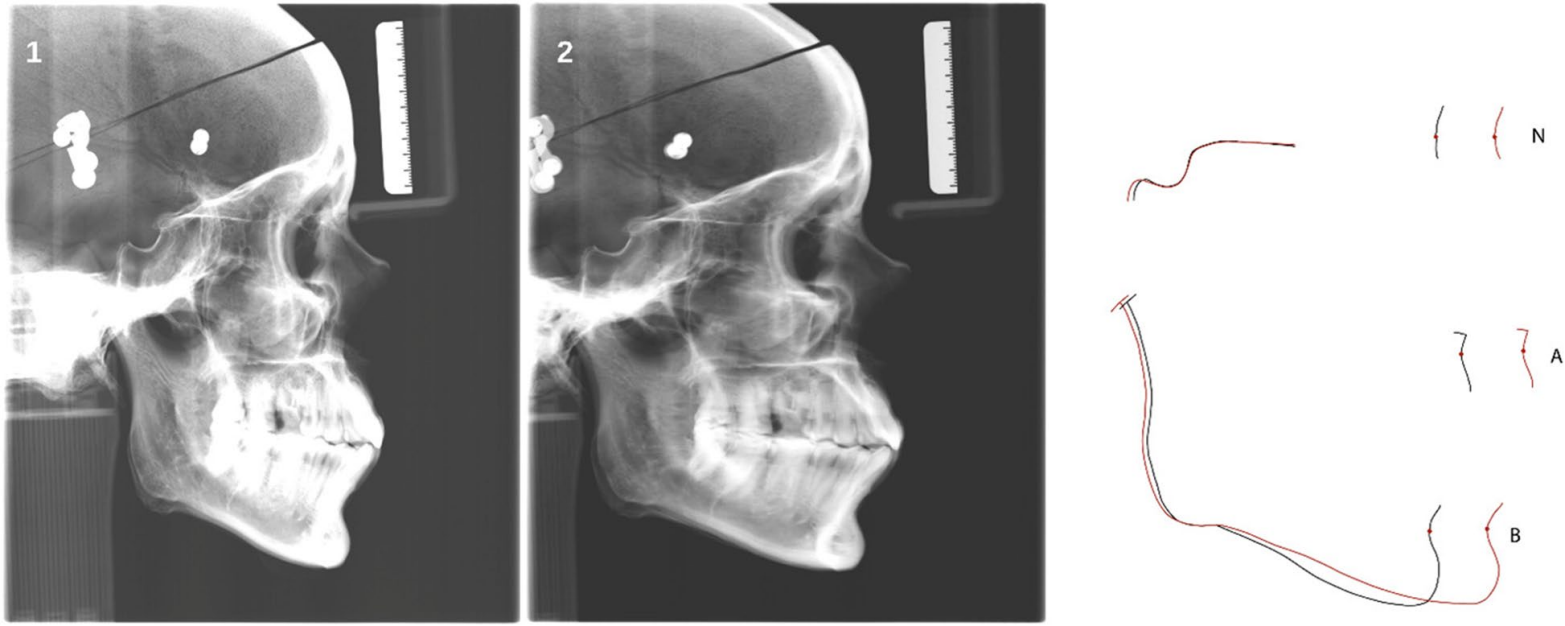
Reference Ceph0 (1) versus Ceph No. 2 (2), where with a dorsal shift of 20 mm was applied. (3) Superimposition of the outlines of the two radiographs (black: Ceph0, red: Ceph No. 2) on the anterior cranial base, showing the large anterior displacement of the points N, A and B as a result of the dorsal shift
